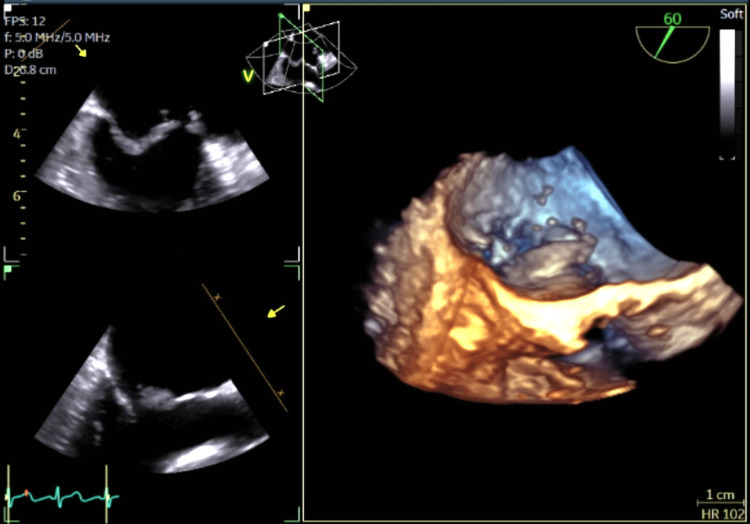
Flail mitral valve orifice demonstrated by 3D post-processing of transesophageal echocardiogram.
